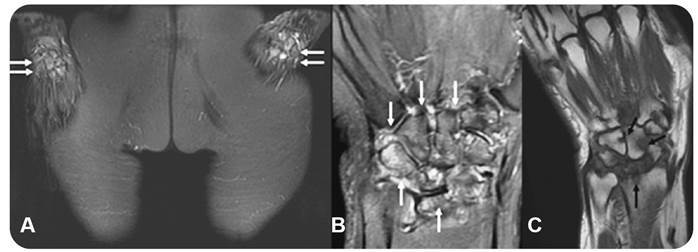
64-year-old female with severe pain in wrists, hands and ankles for two years. Current with no treatment. Coronal STIR (A), coronal PD-weighted (B) and T1-weighted (C) images depict bilateral bone marrow edema of the carpal bones (white arrows) and erosions (black arrows).
